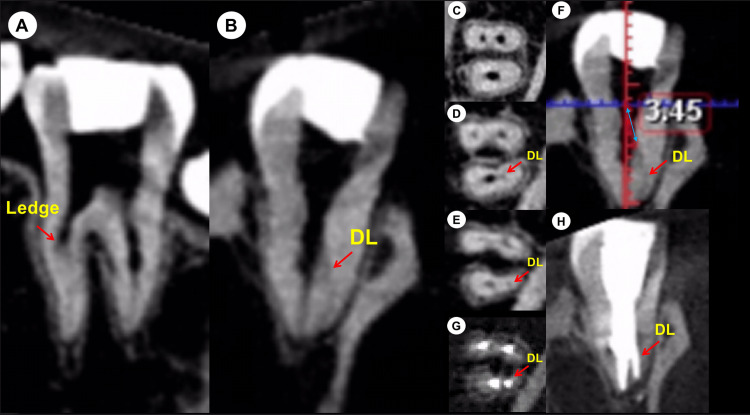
Pre-operative and post-operative CBCT images. (A) The sagittal section of the pre-operative CBCT image displayed a ledge (red arrow) in the mesial canals. (B) The coronal section of the pre-operative CBCT image revealed the emergence of a lingual branch (DL) from the midsection of the distal root canal. (C) The axial section of the pre-operative CBCT image at the coronal third of the tooth did not exhibit a DL canal. (D) The axial section of the pre-operative CBCT image at the middle third confirmed the presence of the DL canal. (E) The axial section of the pre-operative CBCT image at the apical third demonstrated the DL canal. (F) The measurement was taken from the center of the distal canal orifice to the location where the branch emerged from the midsection of the distal root canal (3.45 mm). (G) The axial section of the post-operative CBCT image at the apical third showed that the DL canal was filled. (H) The coronal section of the post-operative CBCT image confirmed that the DL canal was successfully obturated. CBCT, cone-bean computed tomography; DL, distolingual canal
